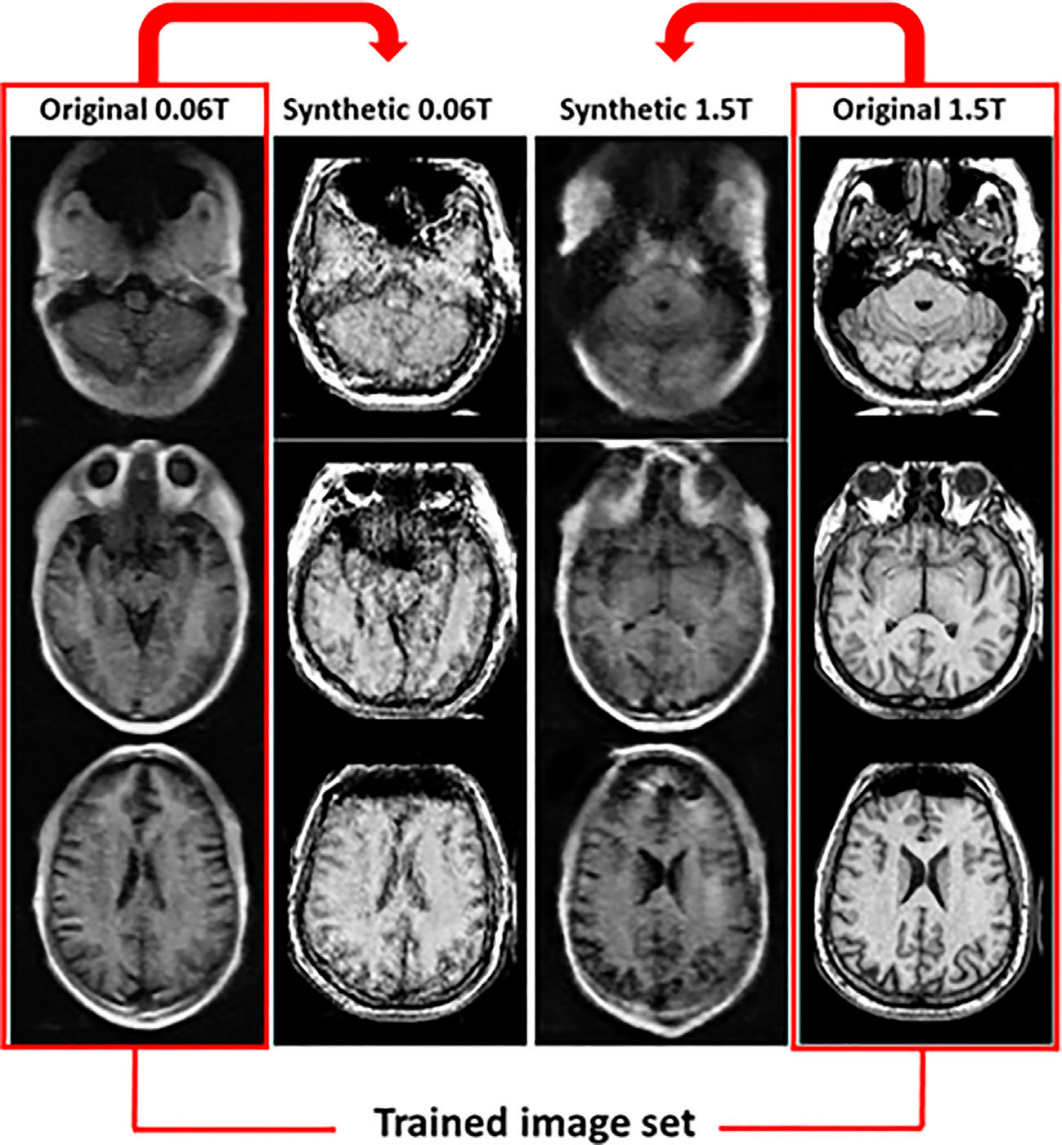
Synthetic MR images from original 0.06 Tesla and original 1.5 Tesla MR images in step 2 (cyclic-GAN model, method 3).
